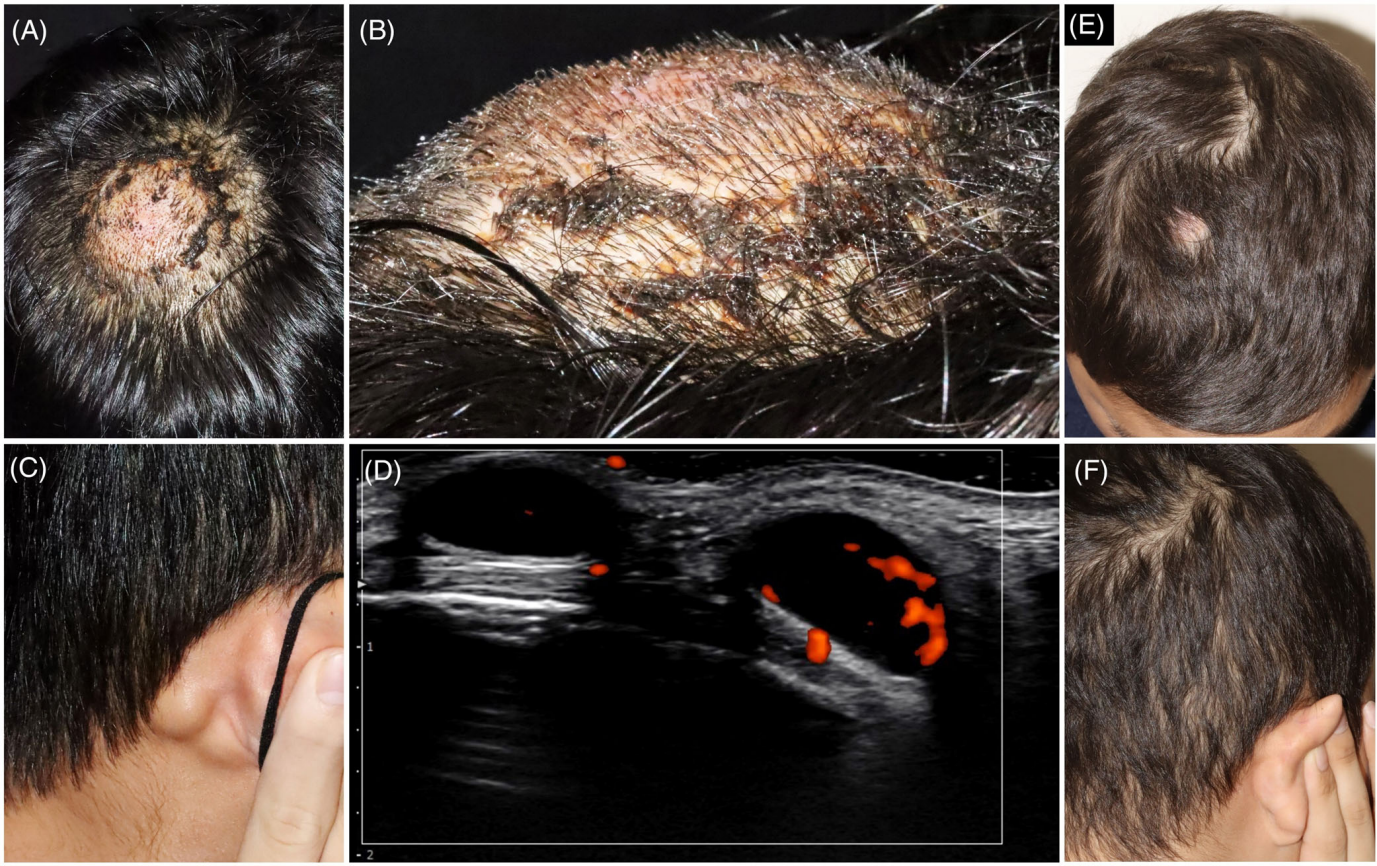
Clinical and ultrasonographic images. (A) A dome‐shaped, firm, non‐tender, erythematous mass, measuring 5 cm in diameter, was located on the right parietal scalp. Mud‐like residues of Chinese herbal ointment were noted on the scalp. (B) Close‐up lateral view of the scalp mass. (C) Enlarged lymph nodes, measuring 1.5 cm, were detected in the right postauricular region. (D) Ultrasonography of lymph nodes revealed two oval anechoic structures with peripheral vascularity in the hypodermis. (E) The scalp mass had completely resolved at the 24‐week follow‐up, leaving only an alopecic patch. (F) The right postauricular lymph nodes exhibited complete resolution at the 24‐week follow‐up.
